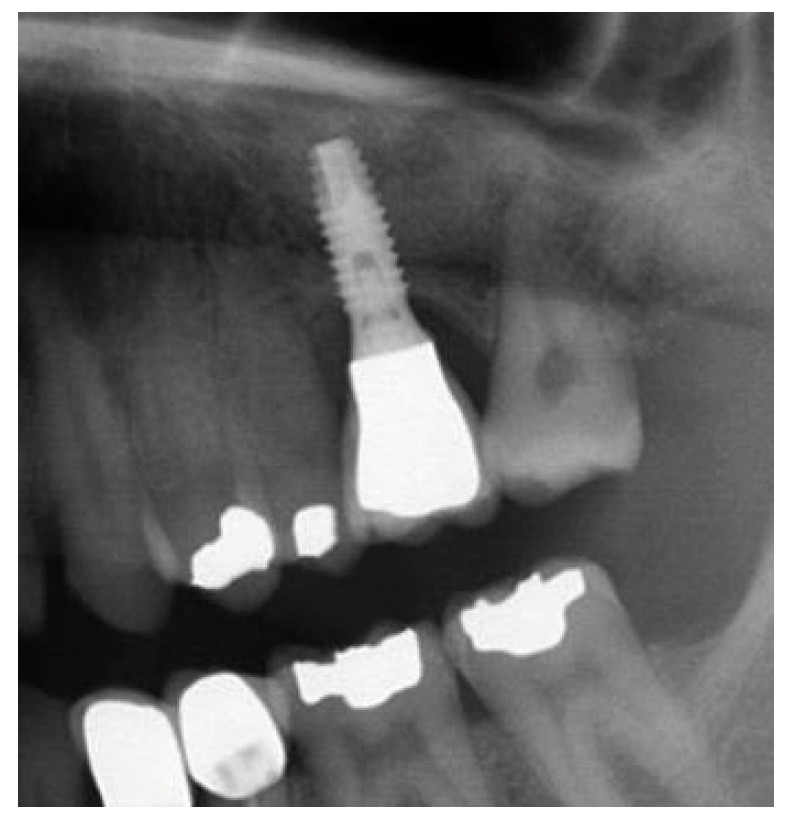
Case 1. 9. Panoramic X-ray view one year after prosthetic loading.
